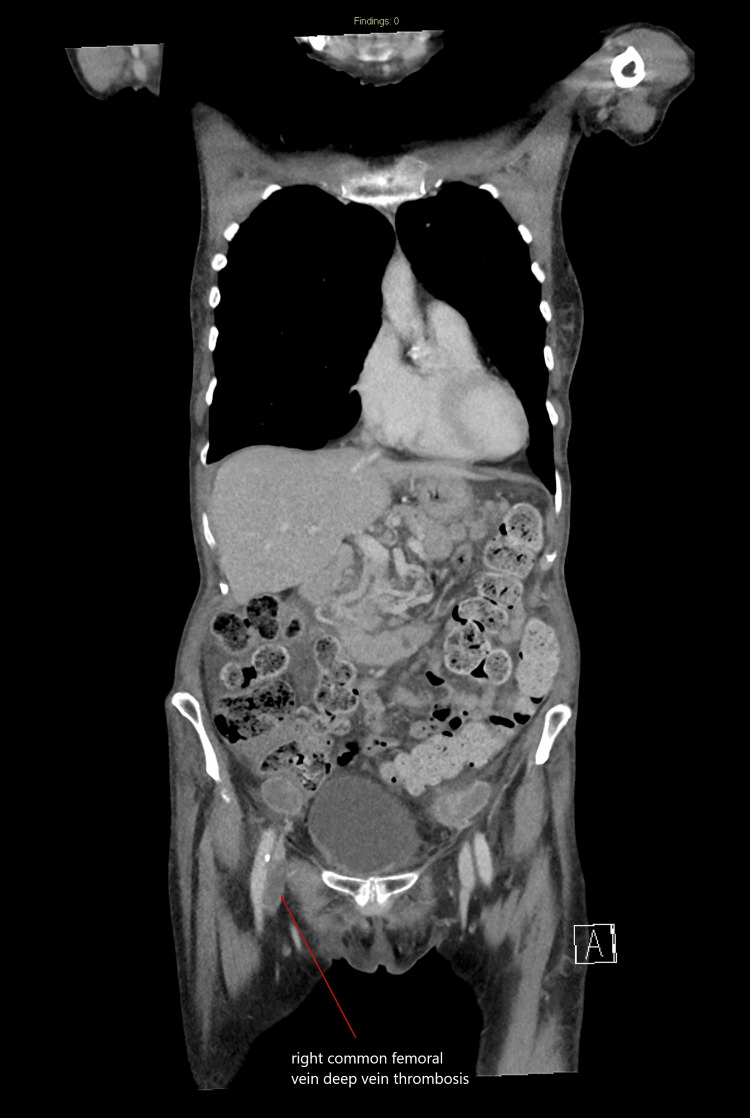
CT of the chest, abdomen, and pelvis with contrast, coronal section, showing a right common femoral vein deep vein thrombosis CT: computed tomography
